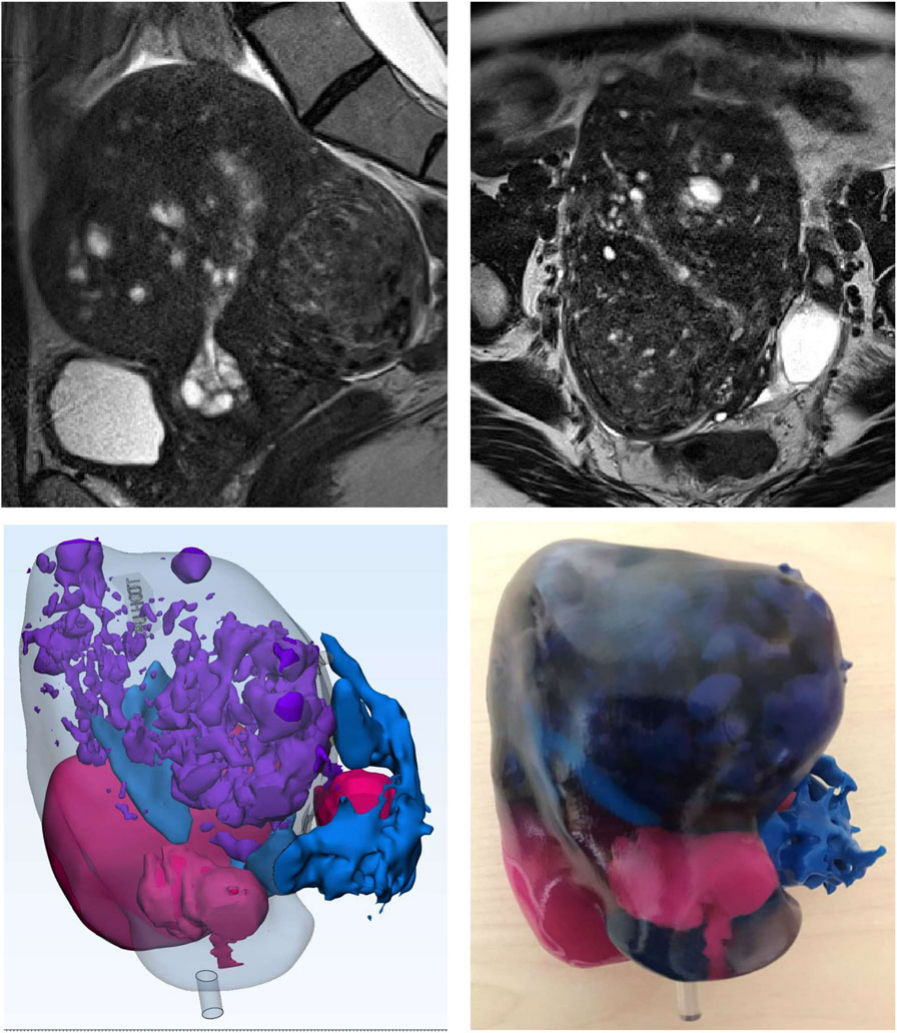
Sagittal (top left) and axial (top right) view of fibroid uterus with adenomyosis depicted on MRI T2 images. MRI images were used to render a 3D digital model (bottom left) and printed as a physical 3D model (bottom right). Adenomyosis was printed in purple, fibroids in magenta, endometrium and external vasculature in blue, and non-neoplastic myometrium and cervical tissue in clear material
